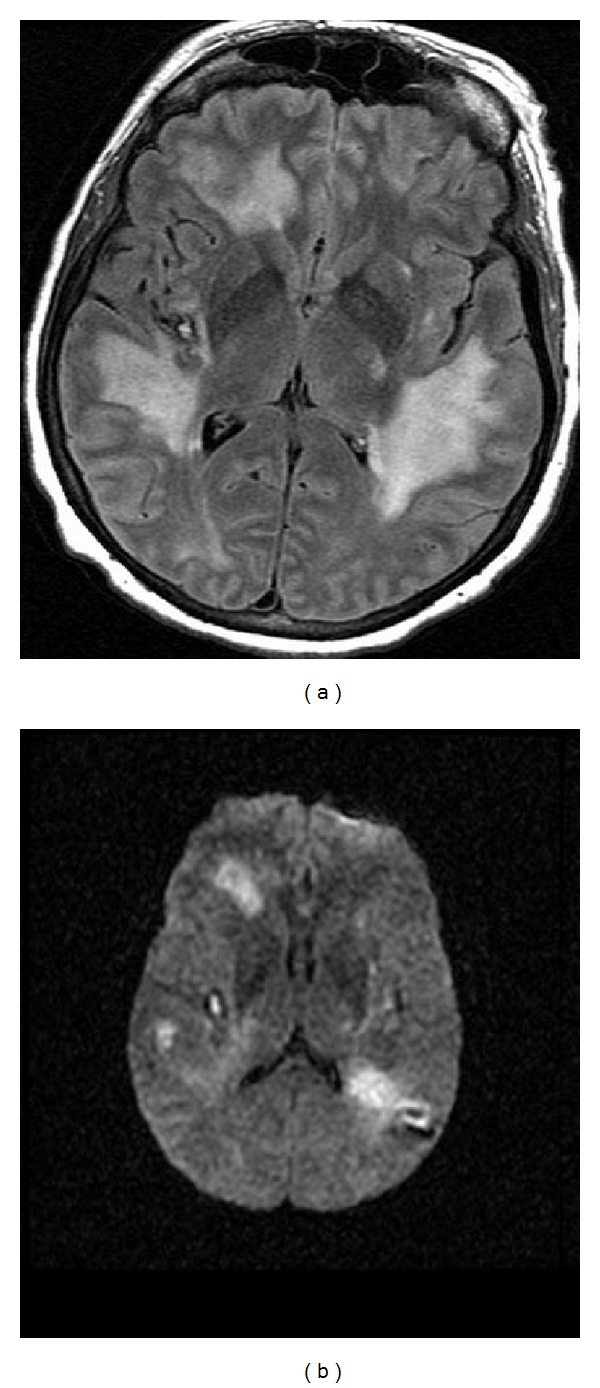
Multiple confluent white matter lesions demonstrated by 3 mm axial fluid attenuated inversion recovery (FLAIR) brain magnetic resonance images (MRIs) demonstrating and diffusion weighted imaging (DWI), respectively.
